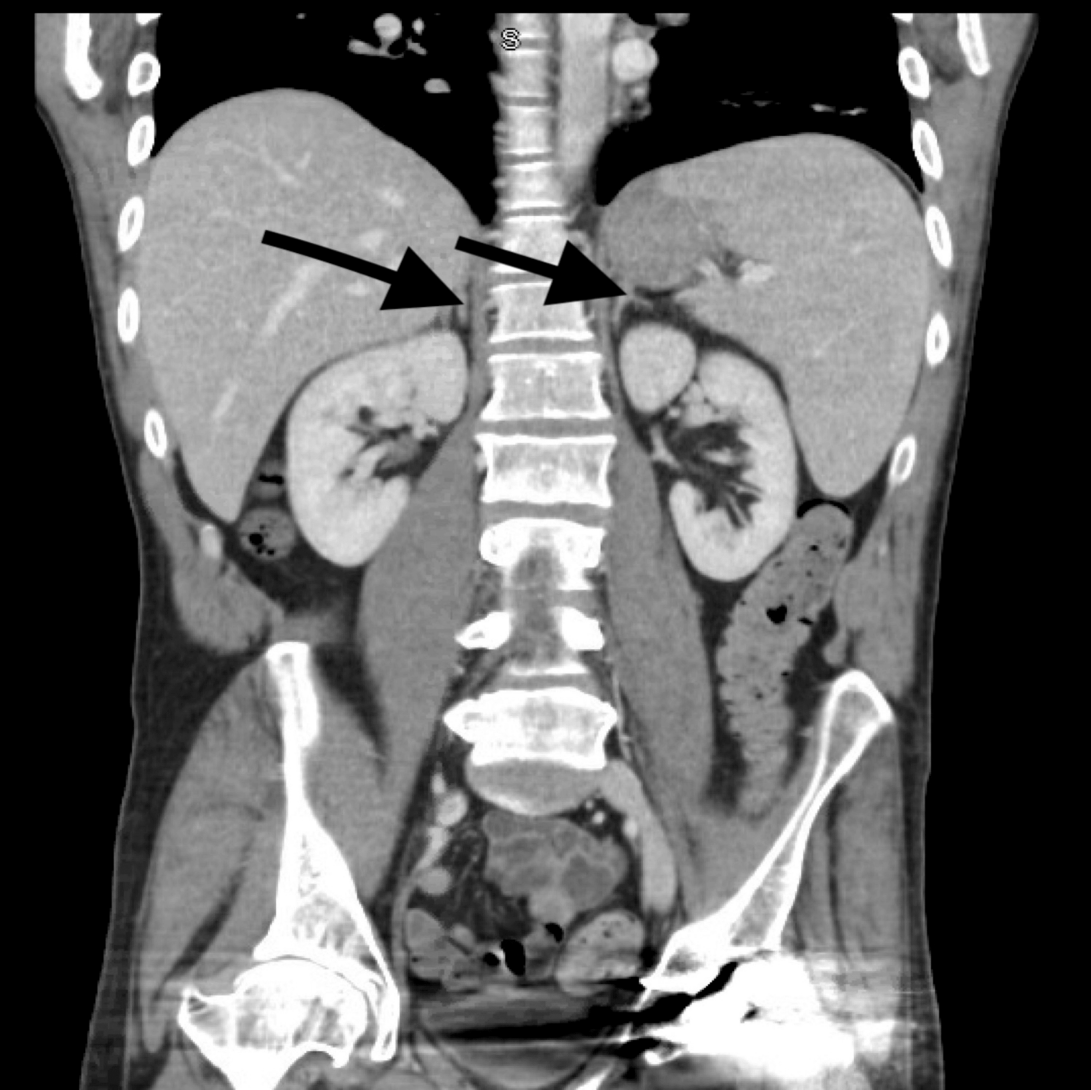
Coronal view of the CT scan of the abdomen and pelvis; arrows indicate normal-sized left and right adrenal glands
